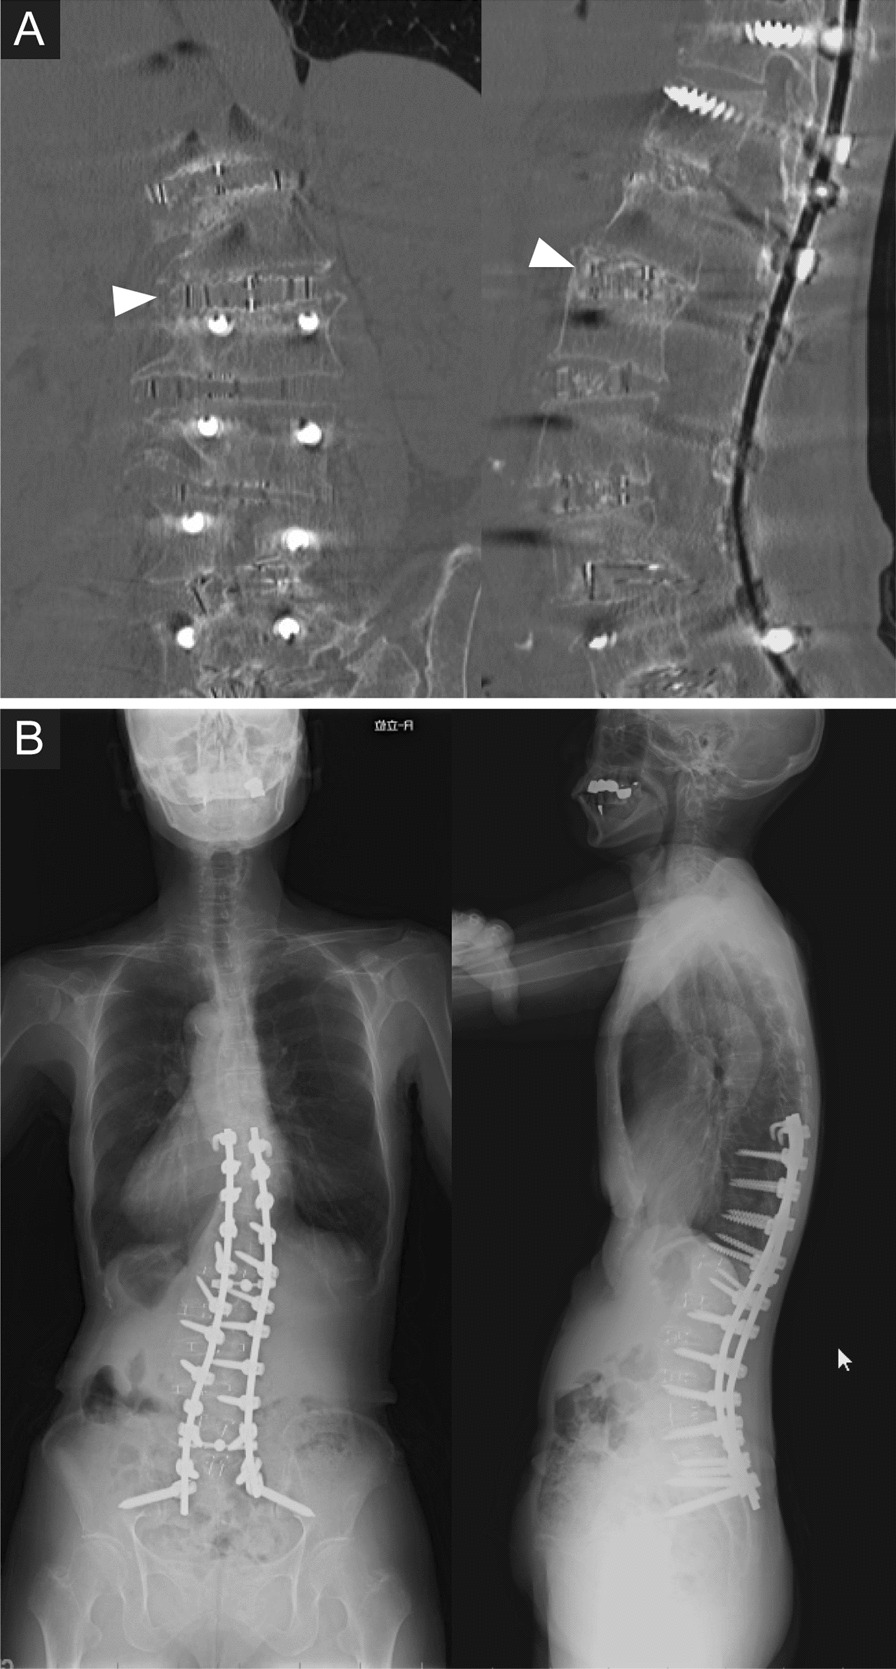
Postoperative images of a 73-year-old woman with rigid kyphoscoliosis treated by two-stage posterior-anterior–posterior surgery. A Postoperative CT demonstrates optimal placement of a lateral lumbar interbody fusion cage at the L1/2 level without endplate injury (arrowheads). B Free-standing posteroanterior and lateral spine radiographs obtained at the 2-year follow-up
